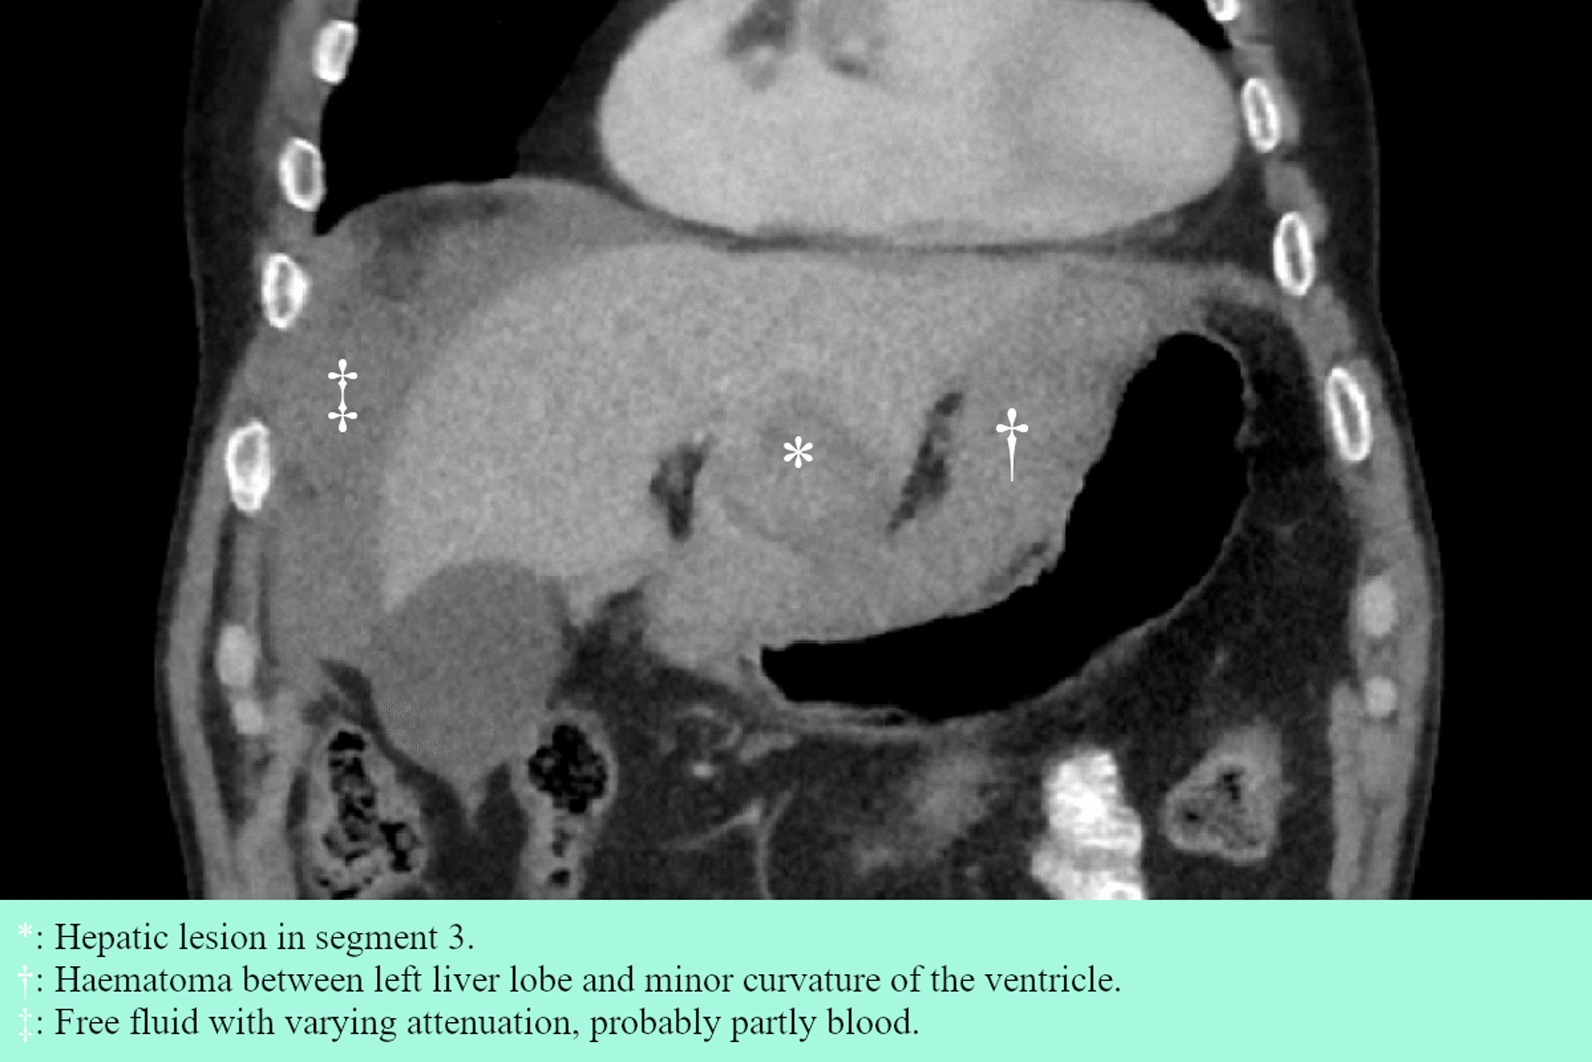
Coronal section of abdominal CT scan on the day of admission
